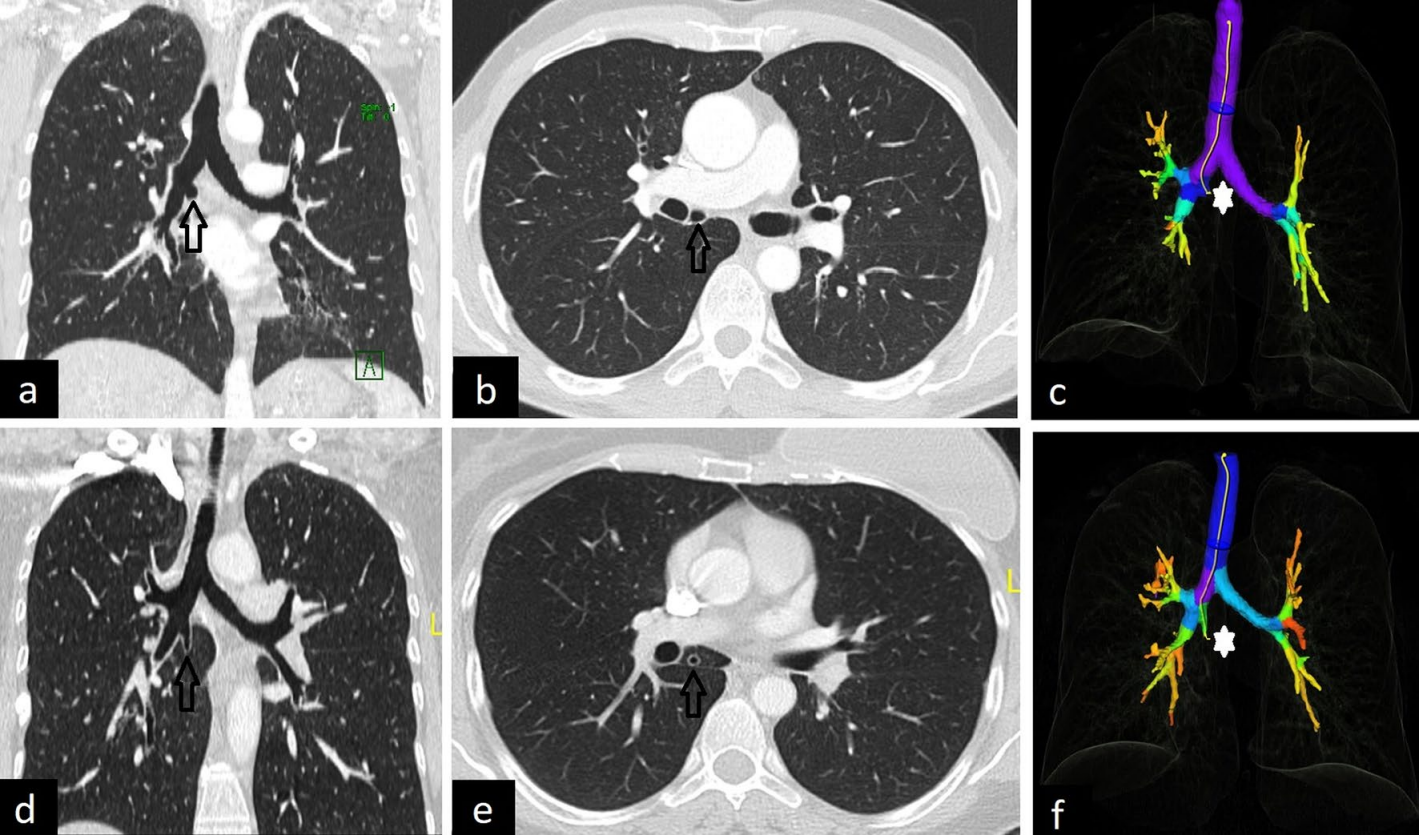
CT images of cardiac bronchi in two patients. Blind-ended cardiac bronchus is seen on coronal (a, arrow), axial (b, arrow) and 3D reconstruction (c, star) CT images. In another patient coronal (d, arrow), axial (e, arrow), and 3D reconstruction (f, star) CT images demonstrate cardiac bronchus with accompanying lung tissue and pleural fissure
